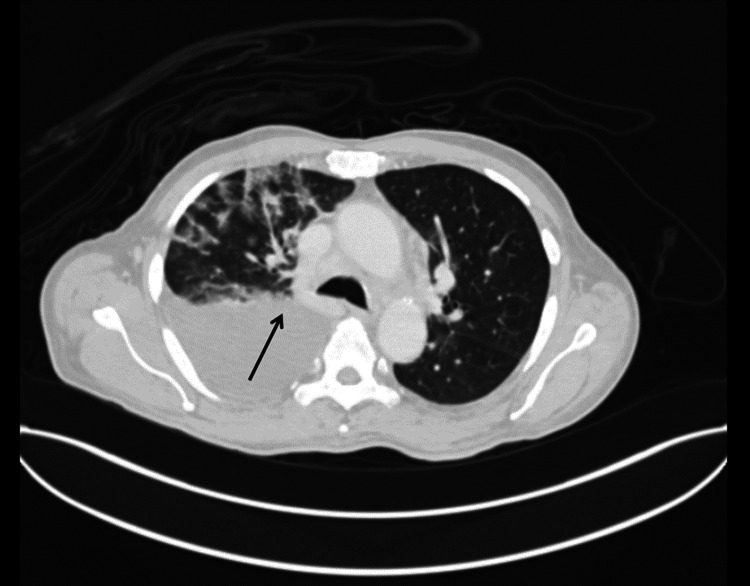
PET CT showing right pleural effusion with pleural thickening
